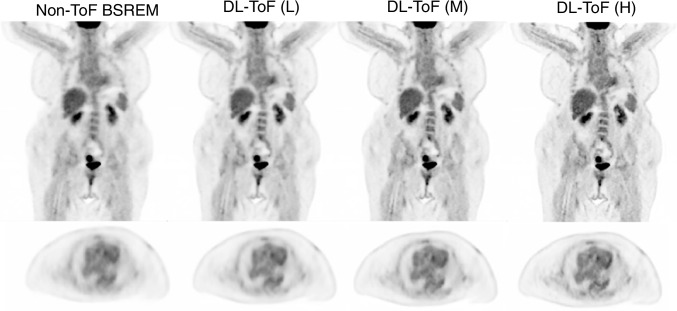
DL-ToF enhancement of a representative test subject with a BMI of 53.8 kg/m^2^ and weight of 93.9 kg with an injected activity of 344 MBq scanned on a GE Discovery IQ non-ToF PET/CT scanner (slice thickness 3.8 mm). Display window: 0–5 SUV
